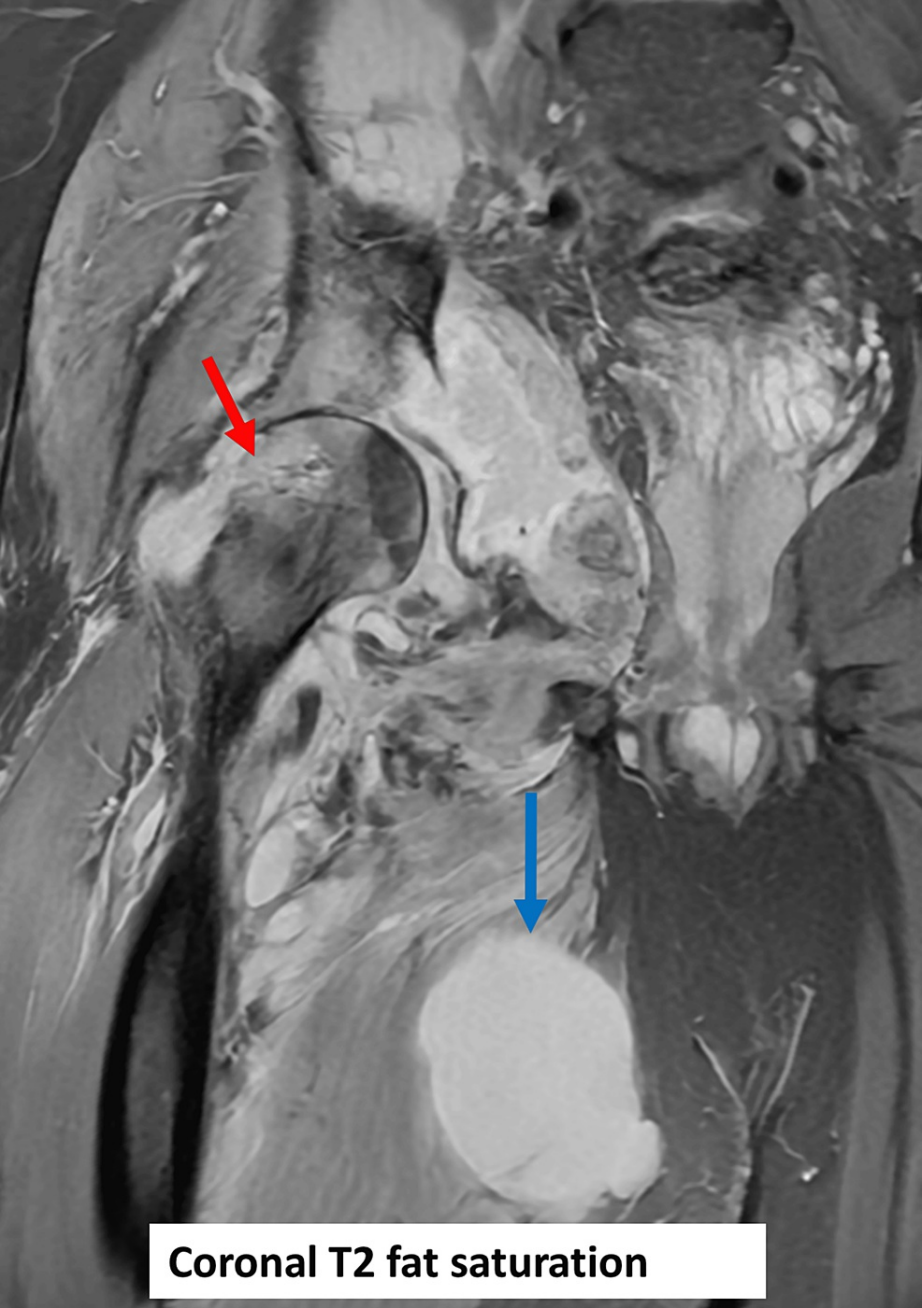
MRI of the right hip with coronal T2 fat saturation. Interval progression of osteomyelitis to the femoral head and neck (red arrow) with a grossly stable appearance of collections distribution with interval increase in the size of adductor collections (blue arrow).
